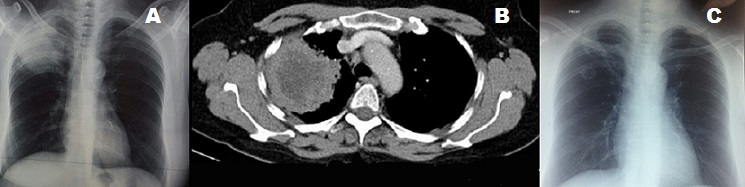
A) chest radiography revealed right upper lobar mass, with spiculated contour; B) chest scanner revealed right upper lobar mass, with spiculated contours; C) chest radiography two weeks after treatment showed resolving pulmonary lesions
